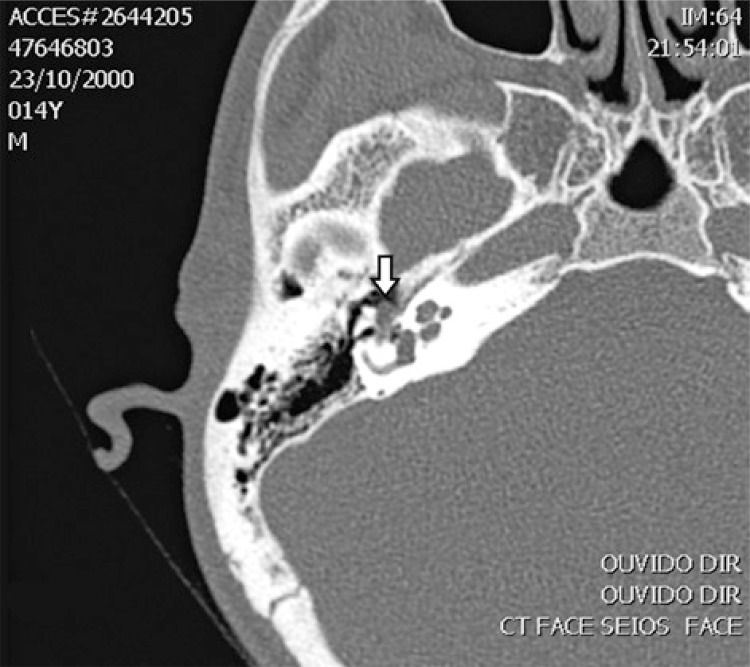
Computed tomography axial section view of temporal bones showing hemangioma (white arrow) of right facial nerve
